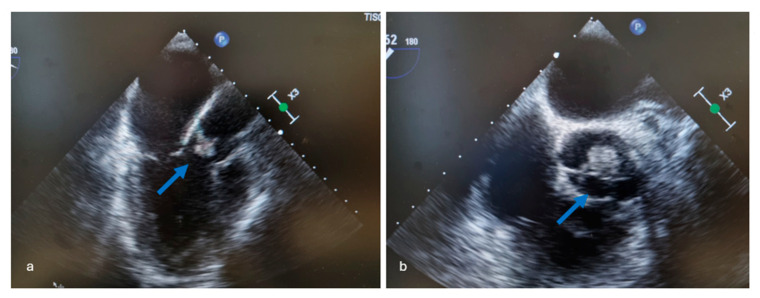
Echocardiographic images of a large vegetation (arrows) due to *Candida* spp. aortic valve endocarditis (a,b).
